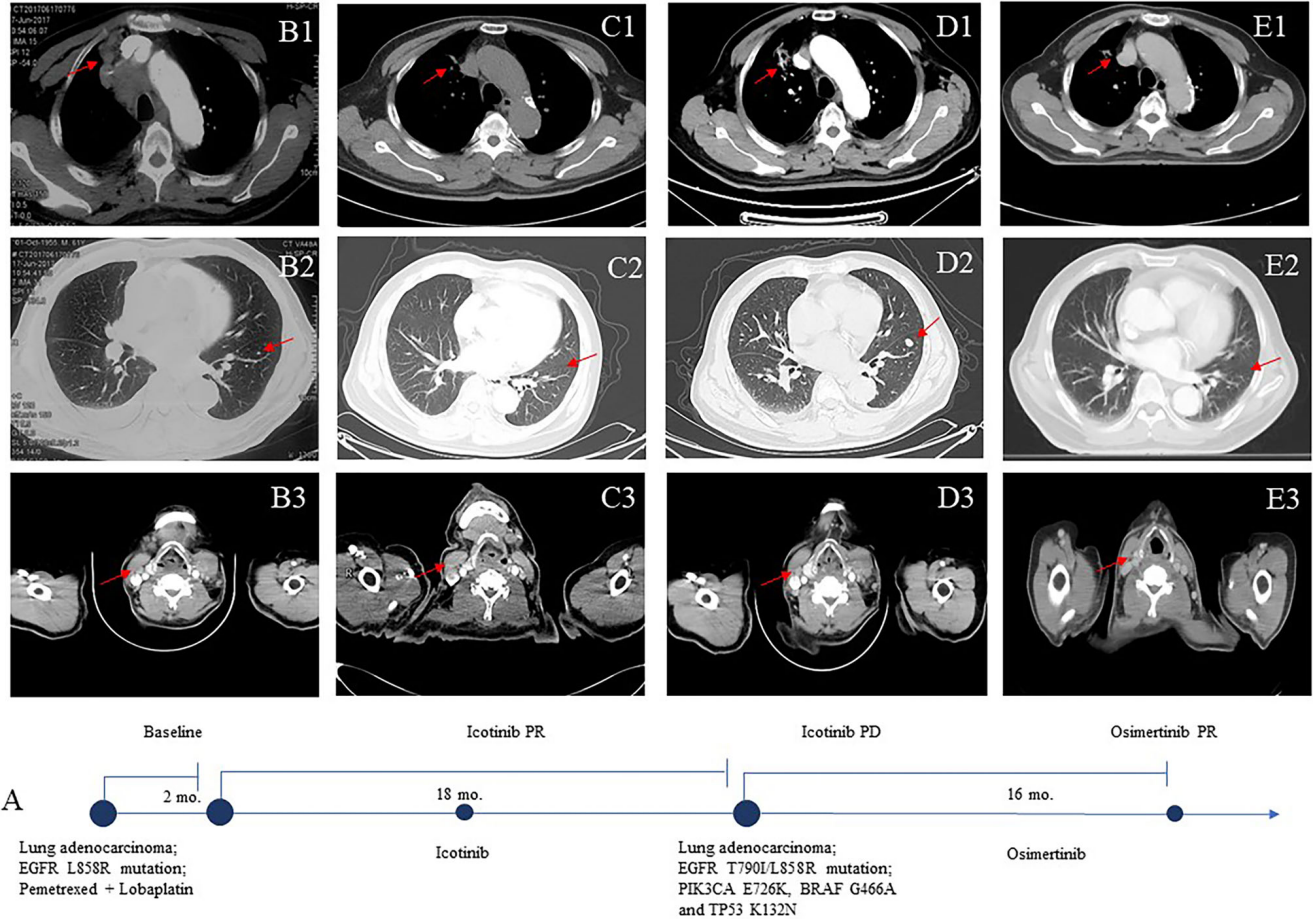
Computed tomographic (CT) images (B–E) of the patient during treatment course (A). Baseline images at diagnosis (June 2017) (B1–3). Images after icotinib treatment (C1–3). The patient’s upper lobe of the right lung, mediastinal and right hilar lymph nodes, bilateral pulmonary nodules, and right supraclavicular region were smaller. Eighteen months after icotinib treatment disease progression (D1–3). The patient achieved a sustainable clinical benefit from osimertinib treatment (E1–3).
